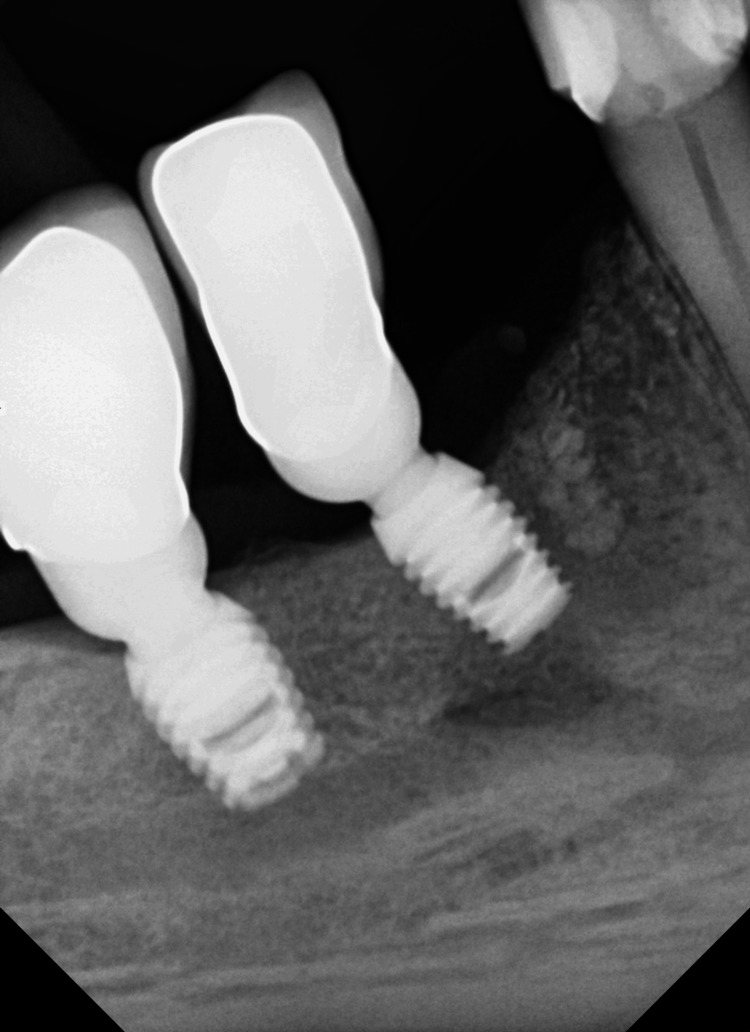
Control X-ray four months post-op
